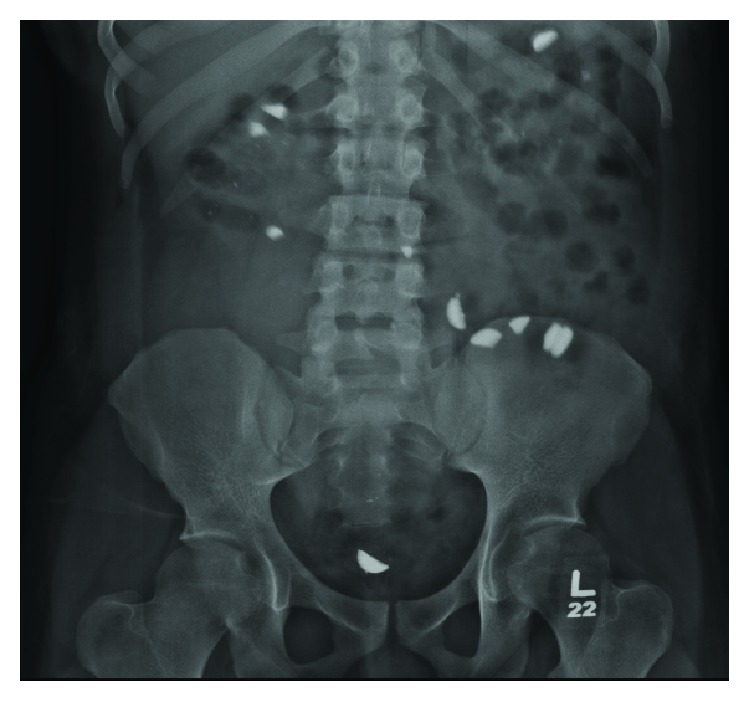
Repeat abdominal radiograph from 6/28/18 shows radiopaque objects moving towards rectum.
